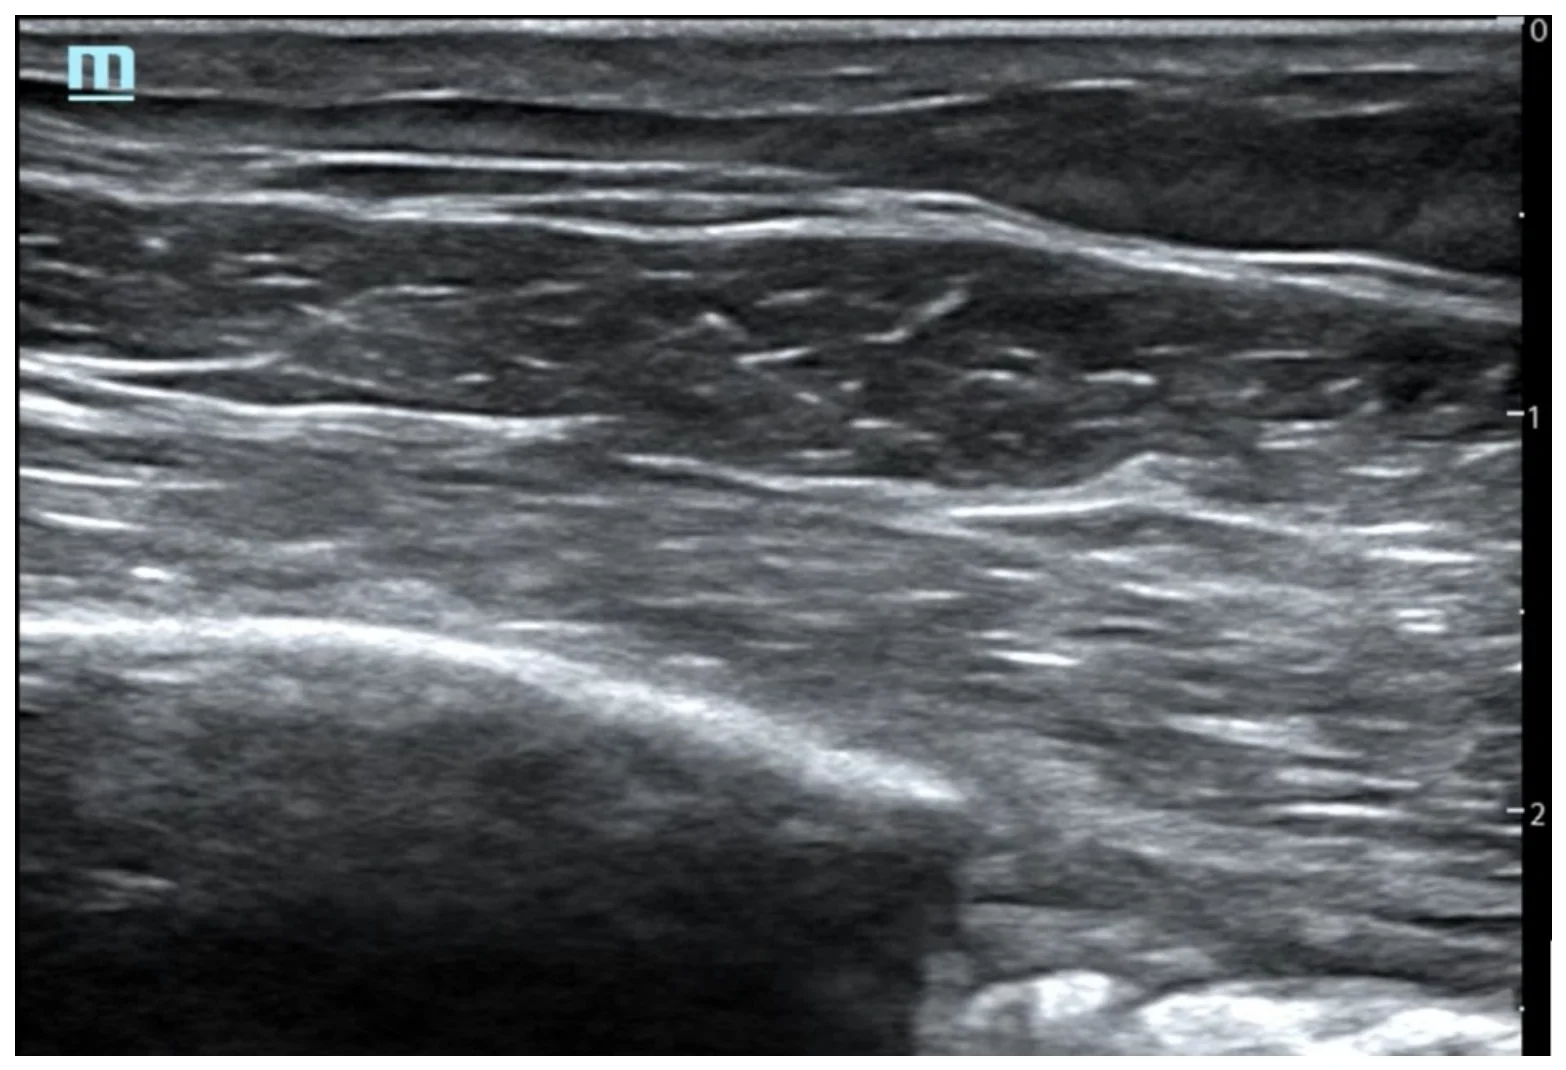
Ultrasound view of pectralis major and minor muscles.
